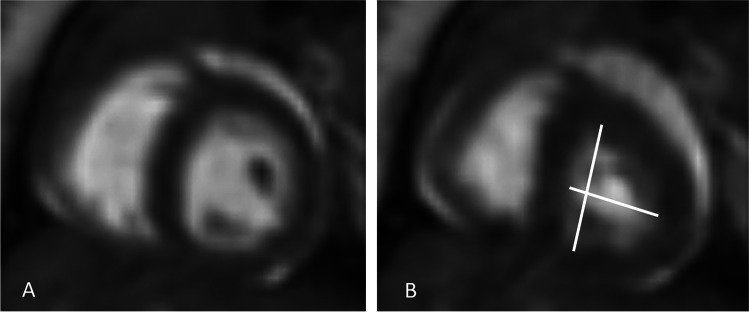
Left ventricular eccentricity index in a boy born at 24 weeks of gestation and imaged at post-menstrual age 40 weeks (16 weeks old). a, b MRI short-axis cine steady-state free precession (SSFP) slice at the level of the papillary muscles shows diastole (a) and systole (b). During systole, the interventricular septum is clearly flattened, creating a D-shape left ventricle. A left ventricular eccentricity index greater than 1.3:1 is diagnostic of pulmonary hypertension
